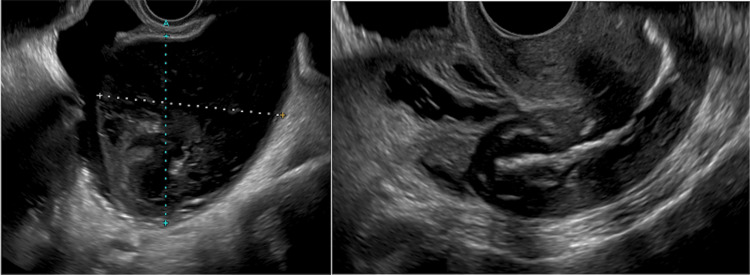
Transvaginal ultrasound Left: An enlarged uterus (77 mm x 78 mm) with internal fluid retention. Right: The uterus after drainage.
